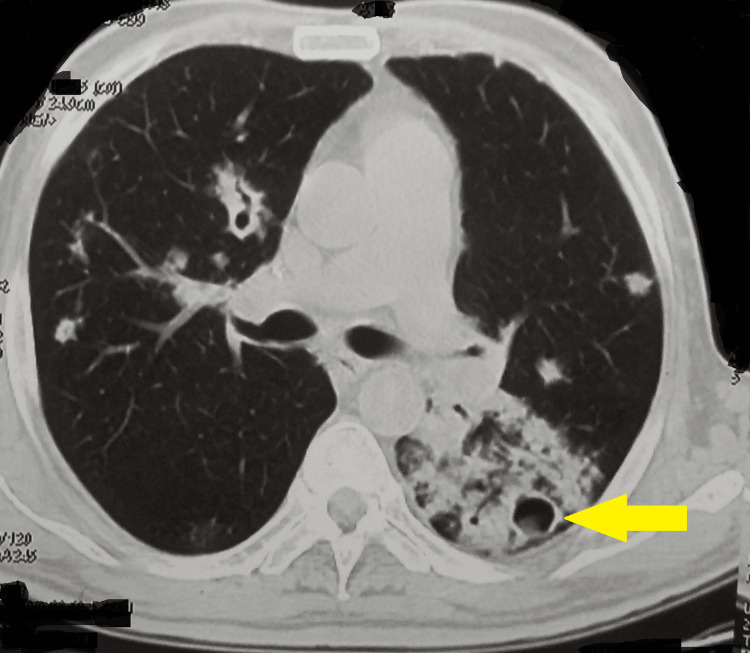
A non-contrast computed tomography of the chest showing a fungal ball with multiple varying-sized cavitary lesions.
